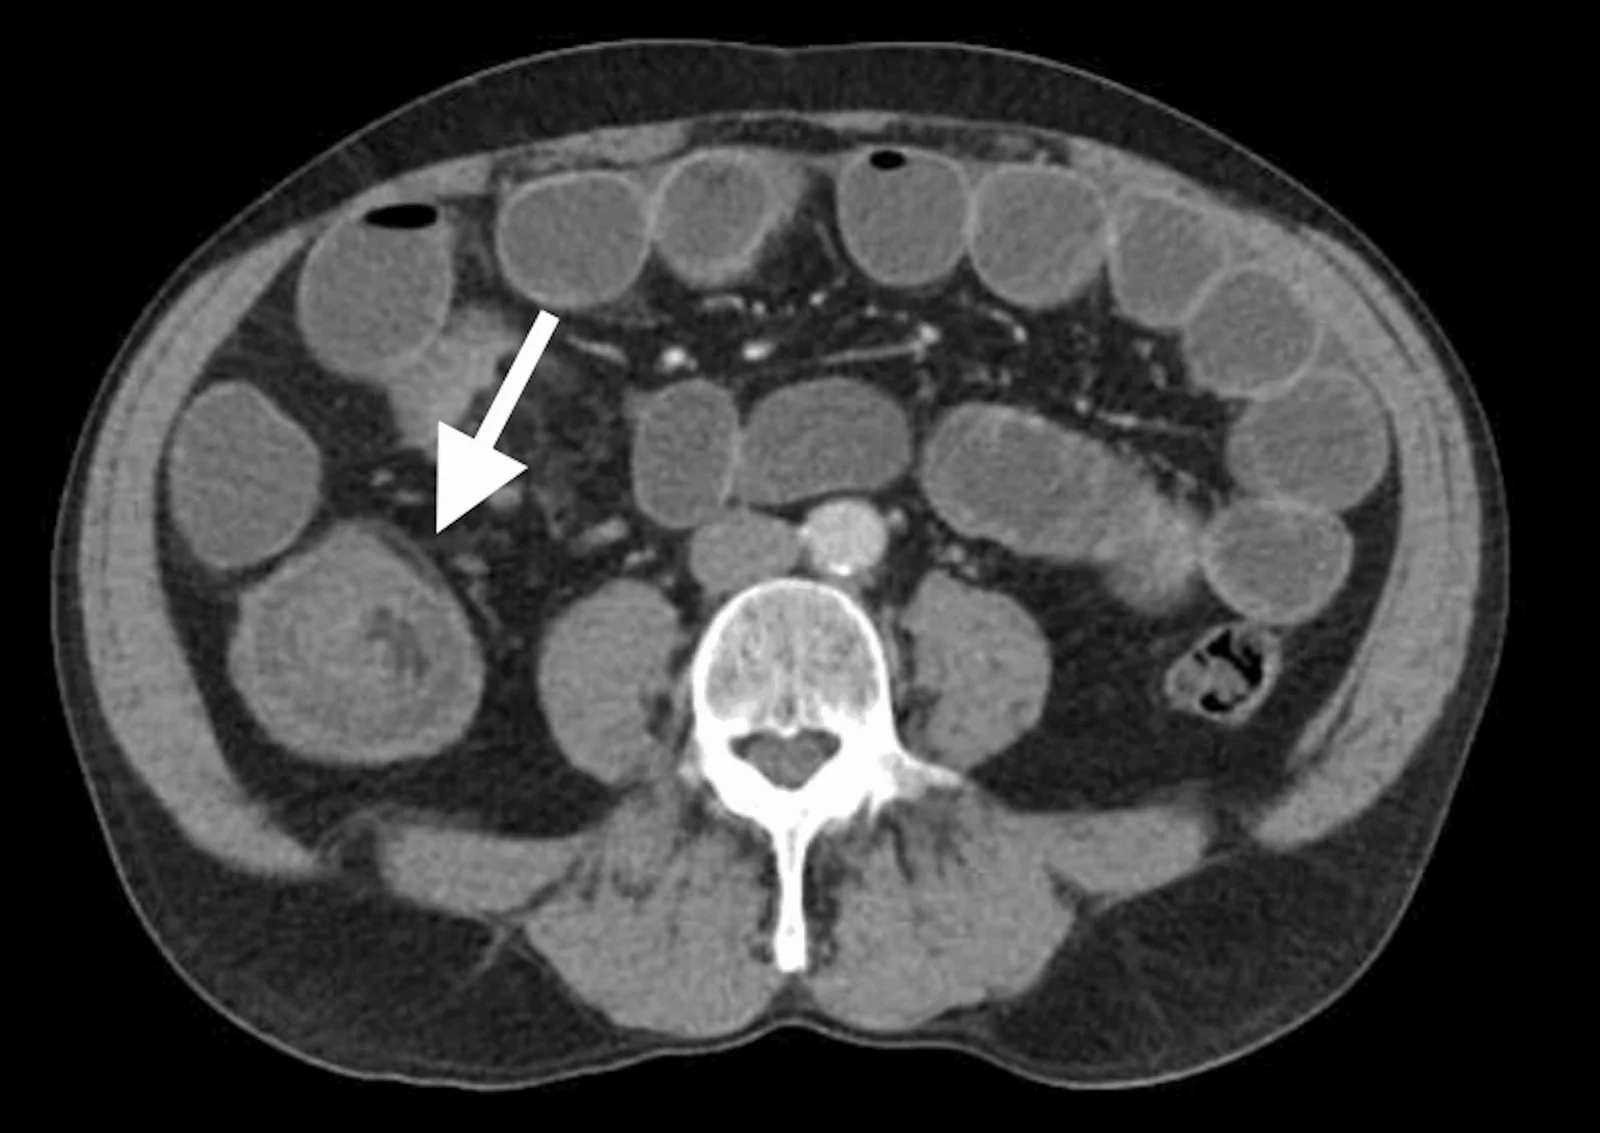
Axial view of the CT abdomen Pathognomonic telescoping ("target sign") is visualized on the left side of the image, as indicated by the arrow. CT, computed tomography
